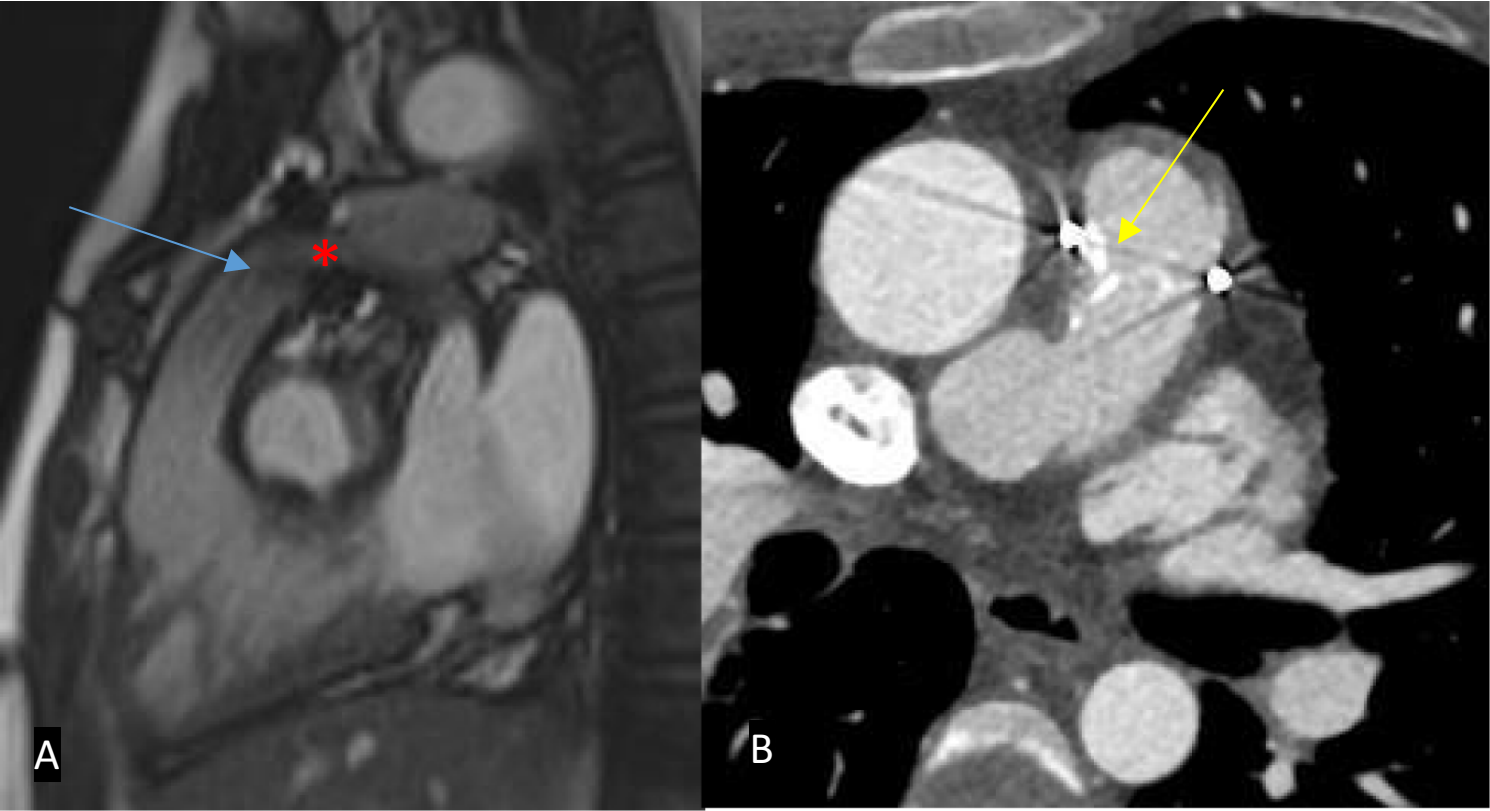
MRI SSFP cine (A) and contrast-enhanced CT (B) images in a patient with a valved (red asterisk) RV-PA conduit. A Single shot of the RVOT demonstrating pulmonary regurgitation (blue arrow). B Single axial image demonstrating calcification around the valve (yellow arrow). RV: right ventricle; PA: pulmonary artery; RVOT: right ventricular outflow tract
